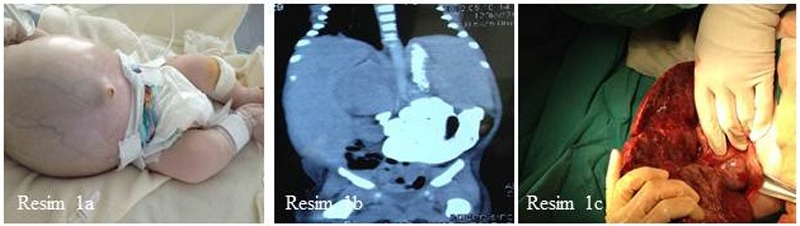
Figure 1: a) Significant abdominal distension with thin venous vessels on abdominal skin was noticed b) computed tomography scan revealed massive hepatomegaly and right adrenal tumor mass c) tumor mass just close to liver and massive hepatomegaly view in operation.
